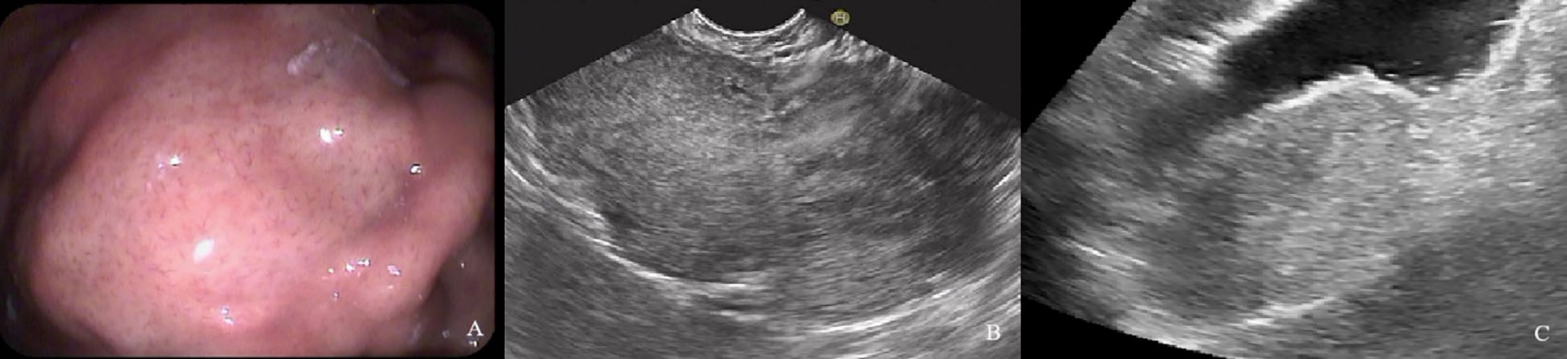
A 60-year-old man with a 9.6×4.9cm GIST of high NIH risk stratification. (A) Gastroscopy showed a submucosal elevation with a smooth surface visible in the gastric fundus. (B) Endoscopic ultrasound showed a hypoechoic mass within the fourth layer of the gastric wall. (C) By oral contrast, ultrasound showed a hypoechoic mass in the fourth layer of the gastric body structure with unclear borders and heterogeneous internal echogenicity.
